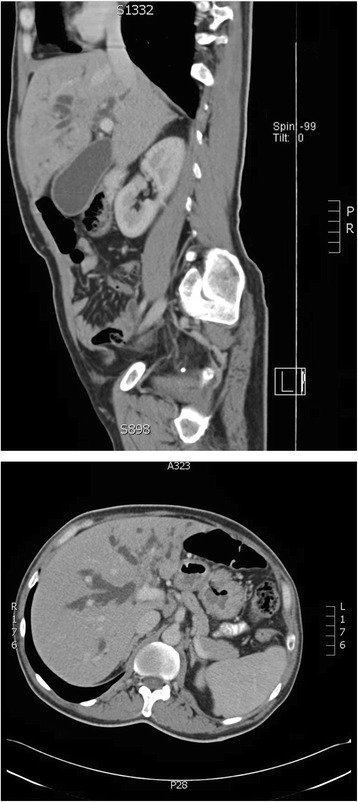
Computed tomography scan of the abdomen, sagittal (*top image*) and axial (*bottom image*) views showing a mass lesion which involved the confluence of the bile ducts extending into both hepatic ducts predominantly affecting the left duct and associated with proximal ductal dilatation
